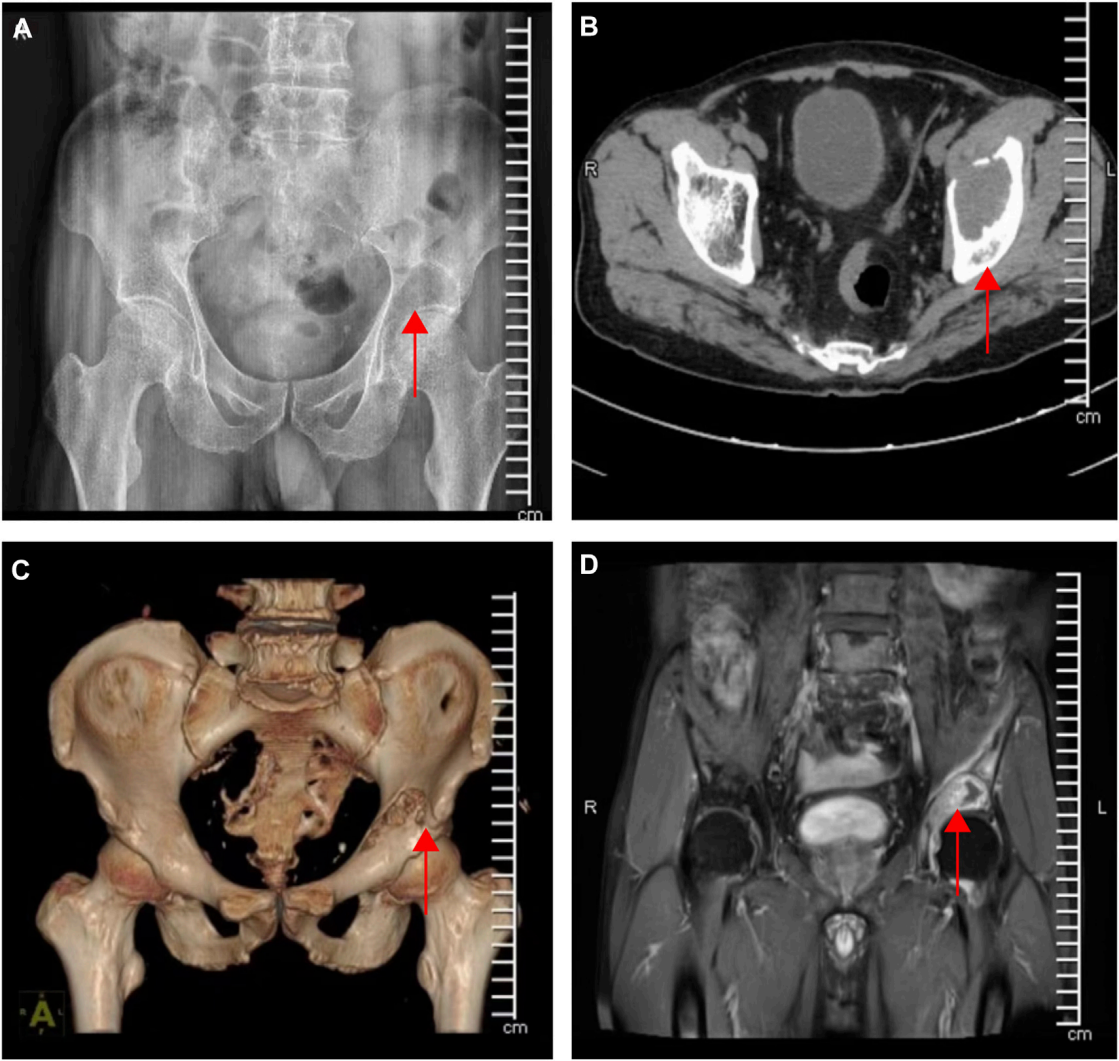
Preoperative imaging data of acetabular metastases. (A) Lesion under X-ray radiographs (B–C) CT and 3D reconstruction of the lesion (D) Magnetic resonance imaging of the lesion (the red arrow indicates the site of the lesion).
